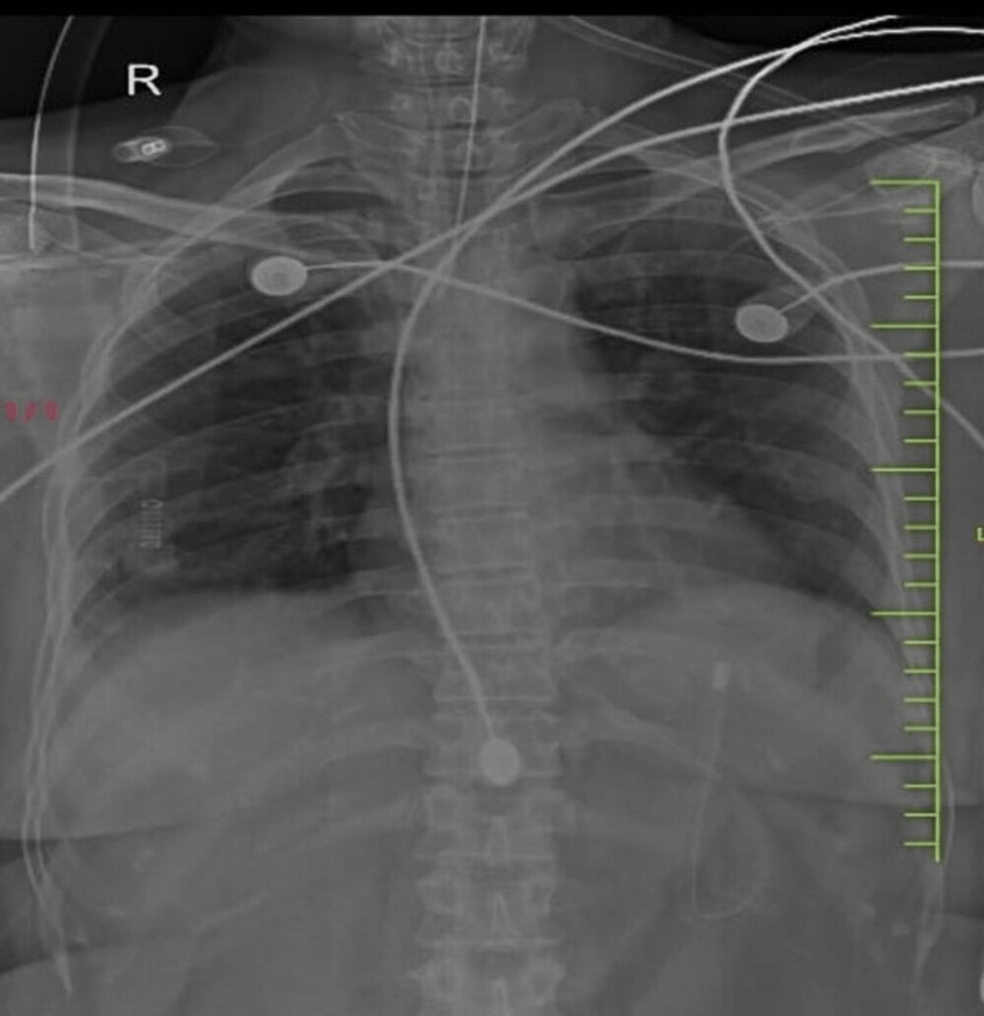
Chest X-ray of the patient showing no obvious abnormality
